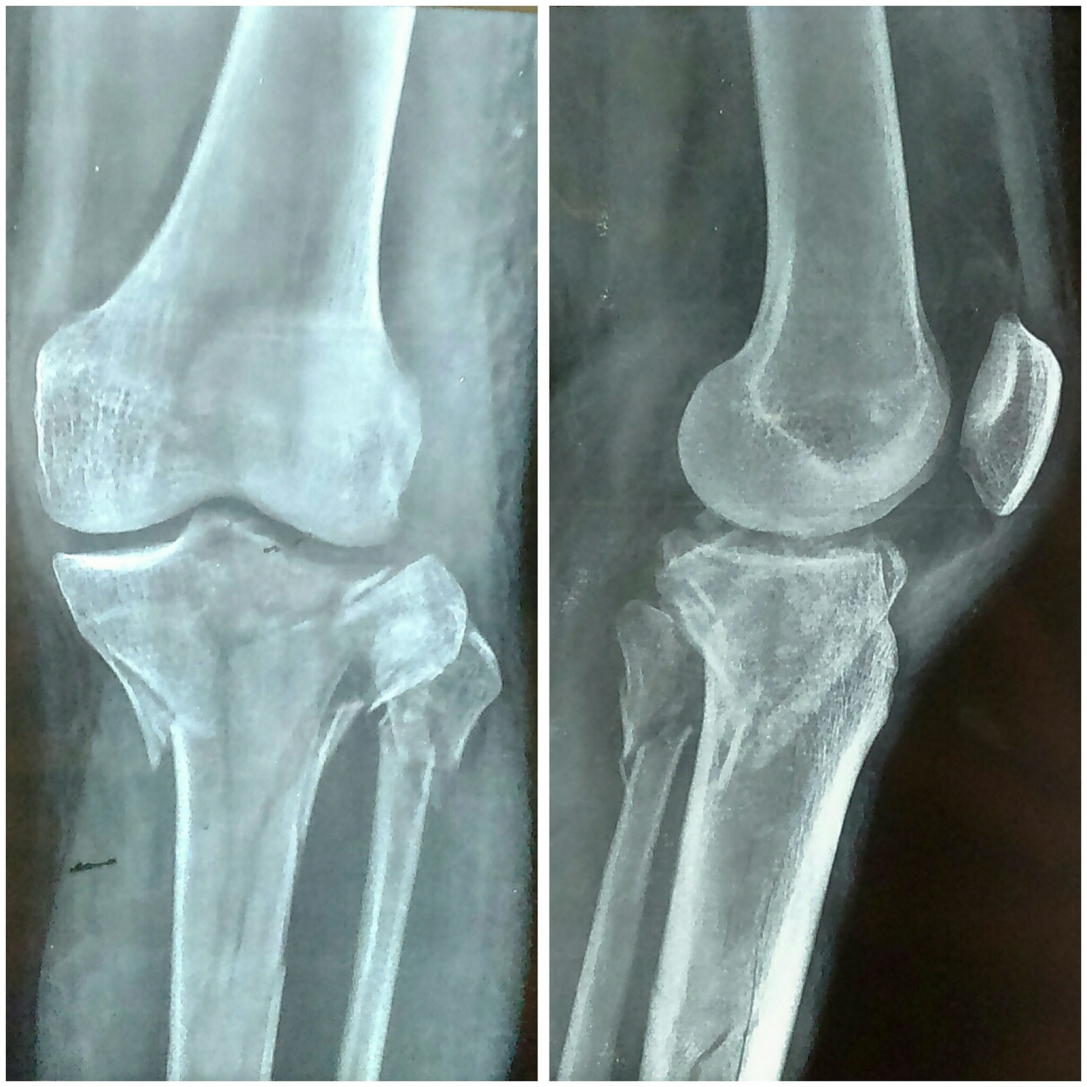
Anteroposterior and lateral X-ray views following injury.
